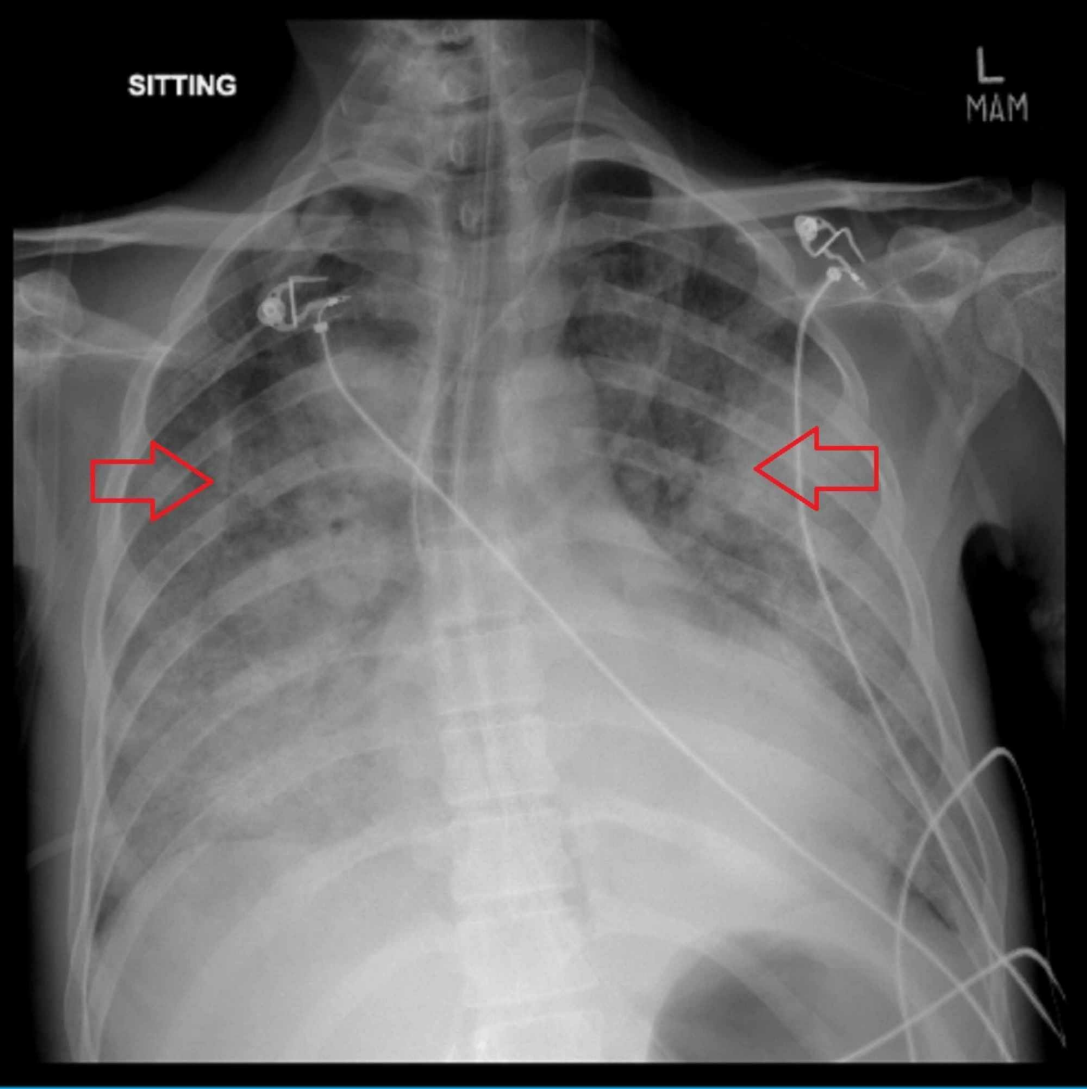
X-ray chest (AP view) showing worsening of pulmonary infilterates (red arrows). AP view: anterio-posterior view.
